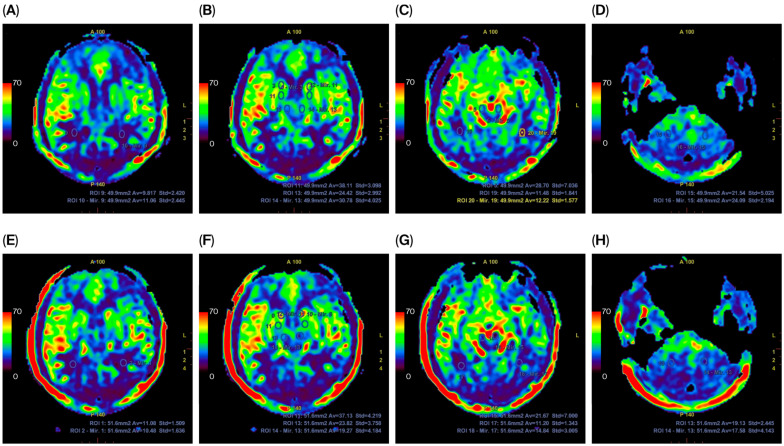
The top row represents pretreatment MRI perfusion (CBF) maps (A–D), and the bottom row represents post-treatment MRI perfusion (CBF) maps (E–H), showing a general increase in CBF. The blue and red areas (bottom and top of the color scale, respectively) indicate the lowest and highest perfusion values, respectively.
